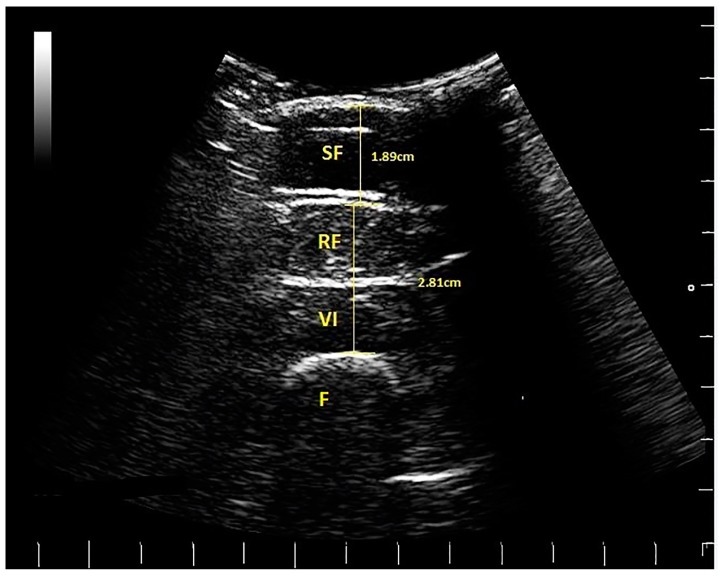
Ultrasound scan from a female golfer, indicating subcutaneous fat (SF), rectus femoris (RF), vastus intermedius (VI) and the femur (F). The scale on the right and bottom of the scan is in cm (white lines). The thickness of the tissues is indicated (SF = 1.89 cm) and muscle (RF plus VI = 2.81 cm).
